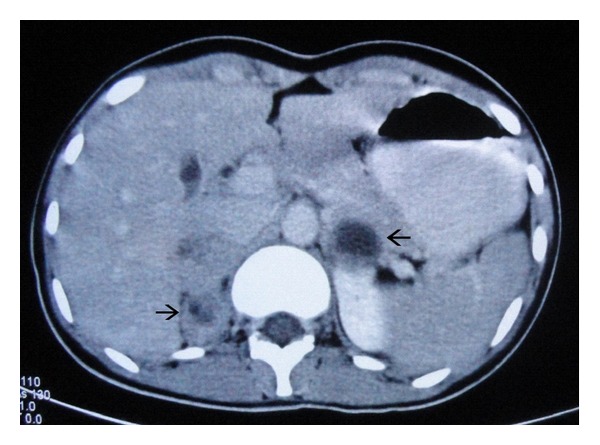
Axial contrast enhanced CT images of abdomen taken at the level of adrenals showing large round heterogeneous lesions in bilateral adrenals with left adrenal lesion showing central necrosis and fluid-fluid level. Central hypodense nonenhancing area is suggestive of necrosis with intense peripheral enhancement.
